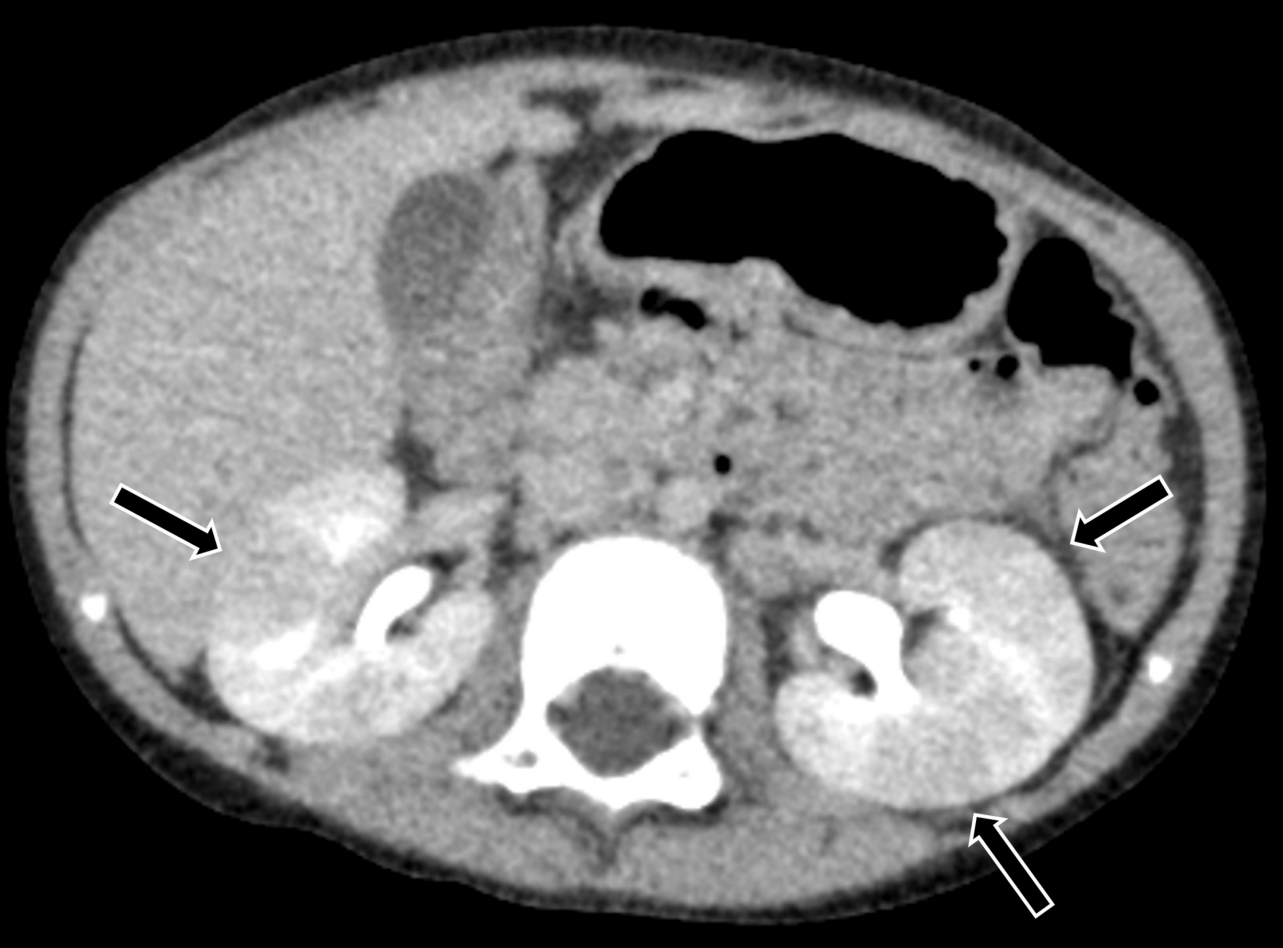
Contrast-enhanced abdominal CT scan showing multifocal hypodense, wedge-shaped space-occupying lesions in both kidneys (arrows)
